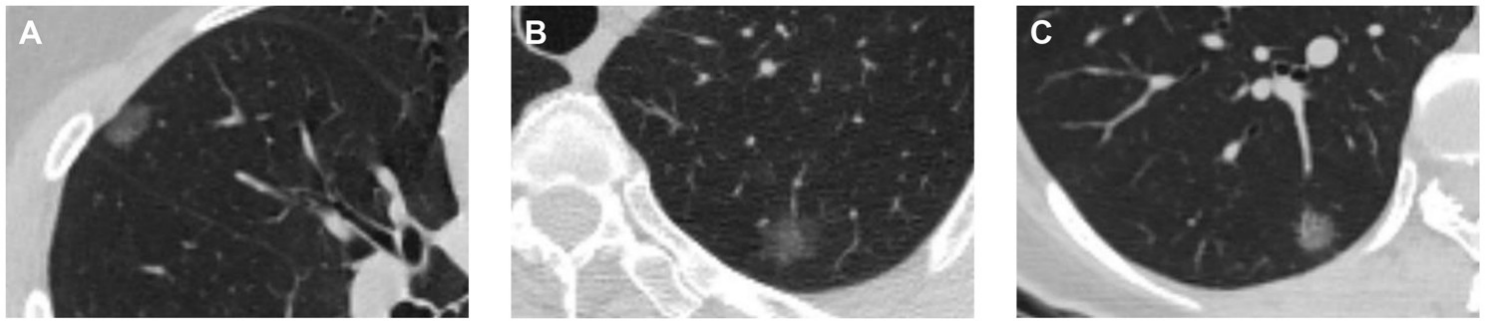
CT images and features of three pure ground-glass nodules with different pathological results. (A) A 39-year-old woman with a pure ground-glass nodule (pGGN) in the right middle lobe was diagnosed pathologically with minimally invasive adenocarcinoma (MIA). The CT image shows that the size of the pGGN is 1.34 cm. The mean CT value of the whole nodule (mCTv) was –694.21 HU, as measured using a region of interest (ROI) after 3D volume segmentation, and the rad_score was –1.340. (B) A 63-year-old woman with a pGGN in the left upper lobe was diagnosed pathologically with lepidic predominant adenocarcinomas (LPA). The CT image shows that the size of the pGGN is 1.55 cm. The mCTv was –708.496 HU, and the rad_score was –1.139. (C) A 42-year-old woman with a pGGN in the right lower lobe was diagnosed pathologically with non-lepidic predominant adenocarcinoma (NLPA). The CT image shows that the size of the pGGN is 1.22 cm. The mCTv was –566.138 HU, and the rad_score was 0.262.
